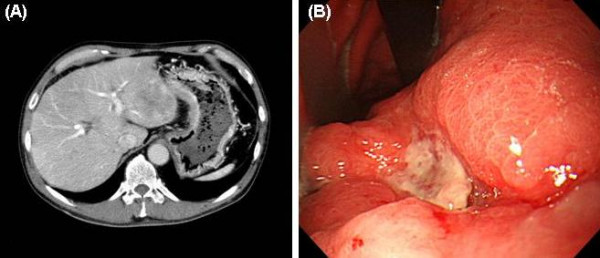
(A) Abdominal computed tomography (CT) revealed enhanced thickness of the gastric wall and multiple liver metastases. (B) Gastroscopy revealed a Borrmann type III tumor on the lesser curvature in the midportion of the stomach.
